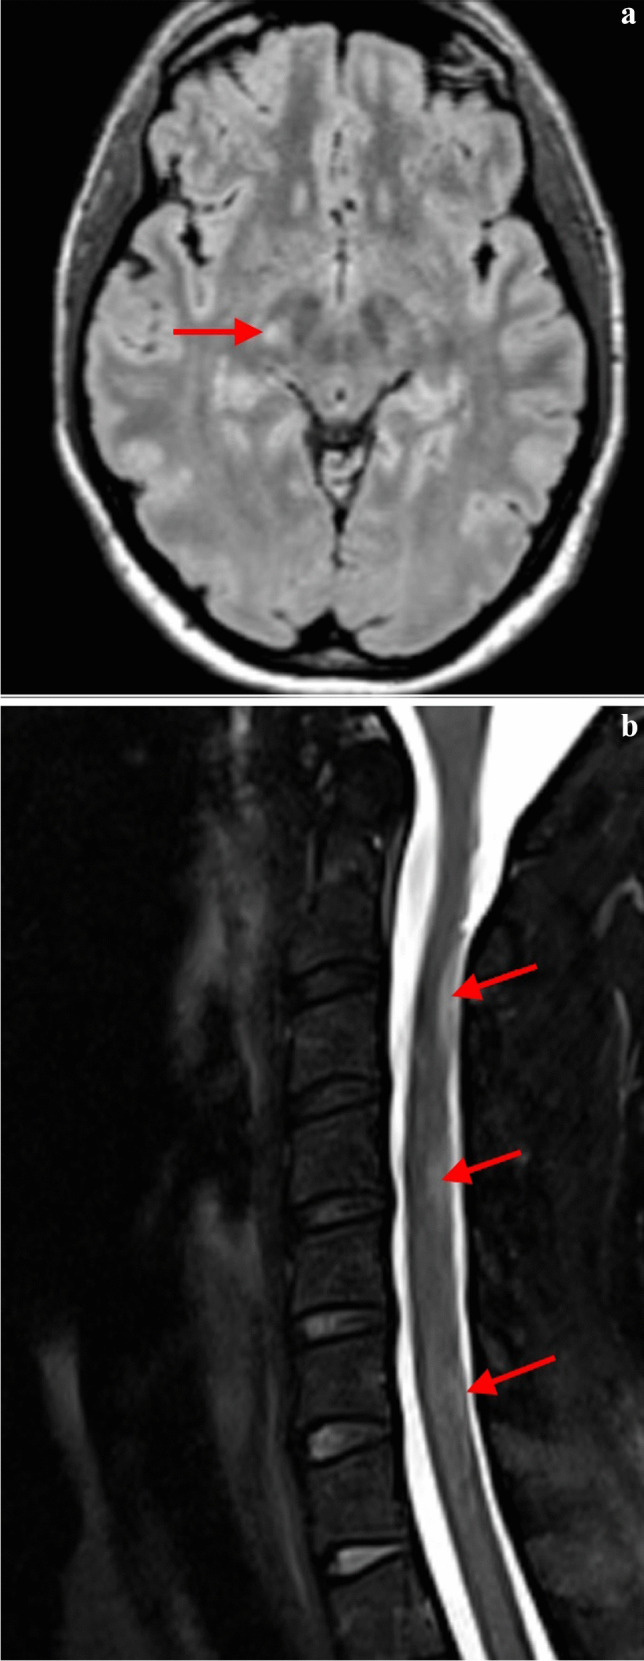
MRI of the patient 2.0. Axial FLAIR shows small lesion (a) and multiple typical laterodorsal hypersignal lesions in cervical spinal cord (b)
